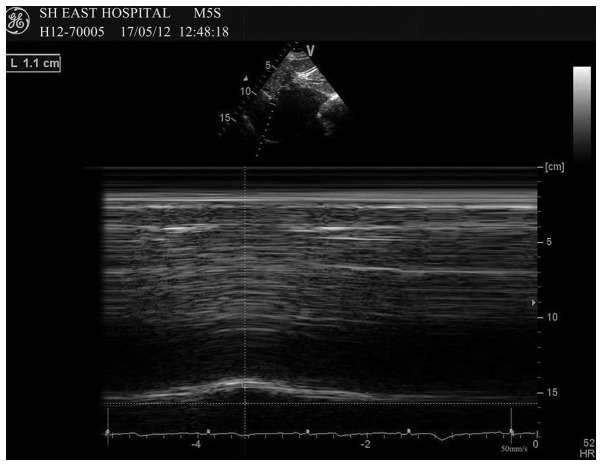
M-mode US of quiet breathing and corresponding cardiac cycle under the hyper-echogenic curve in a healthy subject. US, ultrasound.
